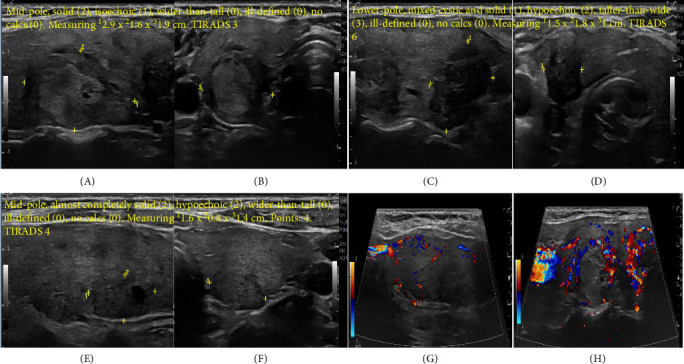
Follow-up thyroid ultrasound after 9 months while patient is clinically and biochemically euthyroid. (A) R mid-pole thyroid nodule in longitudinal axis measuring^1^ 2.94 cm × ^2^ 1.61 cm. (B) R mid-pole thyroid nodule in transverse axis^3^ 1.94 cm. Mid-pole, solid (2), isoechoic (1), wider-than-tall (0), ill-defined (0), and no calcs (0). Measuring 2.9 cm × 1.6 cm × 1.9 cm. Points: 3. TIRADS 3. (C) R lower pole thyroid nodule in longitudinal axis measuring^1^ 1.52 cm × ^2^ 1.8 cm. (D) R lower pole thyroid nodule in transverse axis measuring 3 1 cm. Lower pole, mixed cystic and solid (1), hypoechoic (2), taller-than-wide (3), ill-defined (0), and no calcs (0). Measuring 1.5 cm × 1.8 cm × 1 cm. Points: 6. TIRADS 6. (E) L mid-pole thyroid nodule in longitudinal axis measuring^1^ 1.6 cm × ^2^ 0.8 cm. (F) L mid-pole thyroid nodule in transverse axis measuring ^3^ 1.4 cm. Mid-pole, almost completely solid (2), hypoechoic (2), wider-than-tall (0), ill-defined (0), and no calcs (0). Measuring 1.6 cm × 0.8 cm × 1.4 cm. Points: 4. TI-Rads: 4. (G) L thyroid doppler in longitudinal axis with normal vascularity. (H) R thyroid doppler in longitudinal axis with hypervascularity more than the L side.
